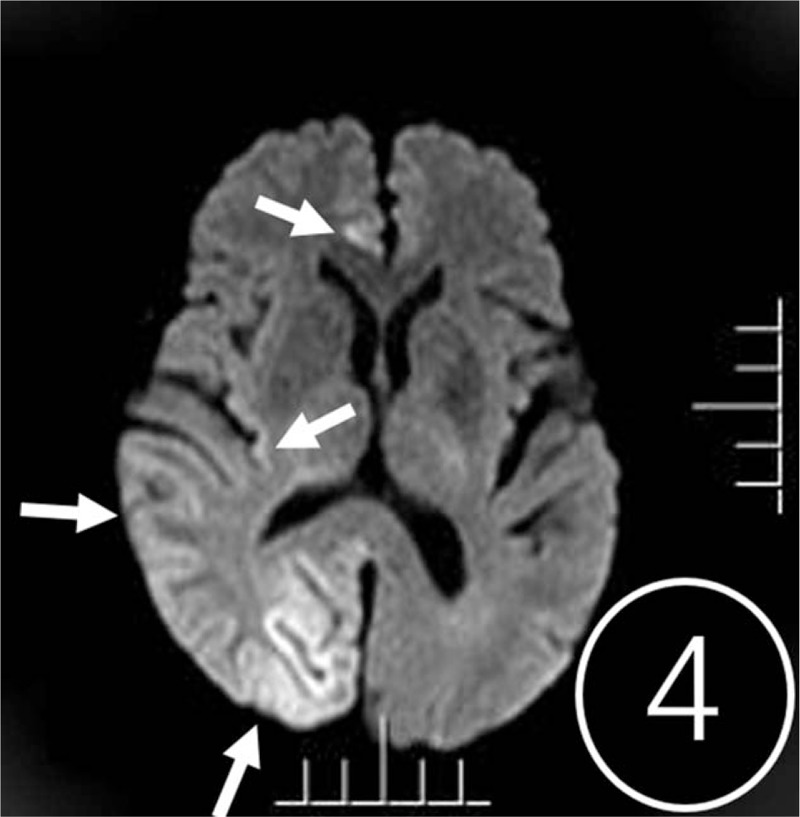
Diffusion-weighted imaging (DWI) showed multiple regions of the cortical/subcortex displayed hyperintensity in the right hemisphere (arrow).
